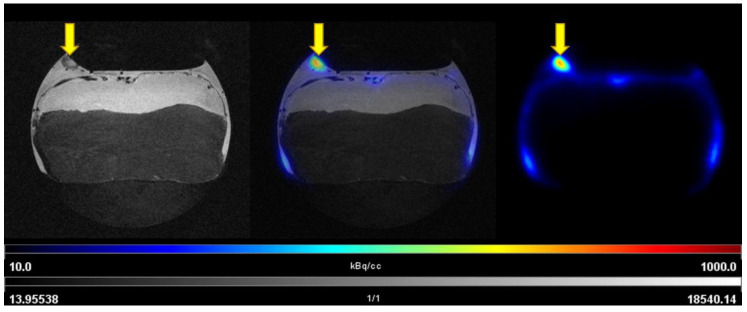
Representative images of 2-[^18^F]FDG PET/MRI on EDD18 using the HT29 CAM-xenograft model. PET imaging was performed 60 min after injection of 8.94 MBq 2-[^18^F]FDG into a CAM-vessel on EDD18. Left: T2-weighted TurboRARE image, right: PET, middle: fused PET and MR (axial planes are shown).
